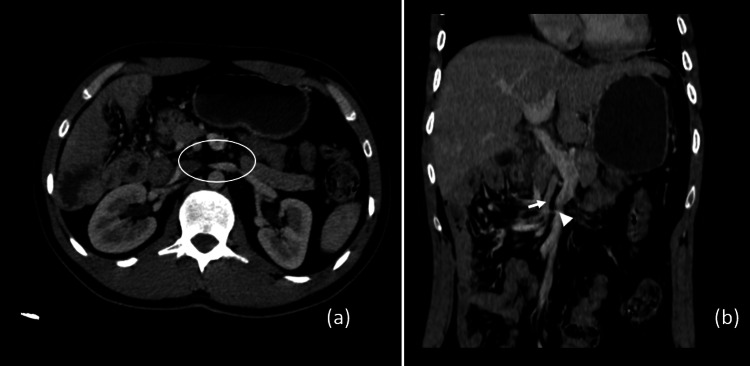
CT images demonstrating (a) the absence of the third portion of the duodenum in the aorto-mesenteric clamp and (b) the abnormal superior mesenteric artery-superior mesenteric vein (SMA-SMV) relationship associated with intestinal malrotation, with the SMV (arrowhead) being to the left of the SMA (full arrow).
